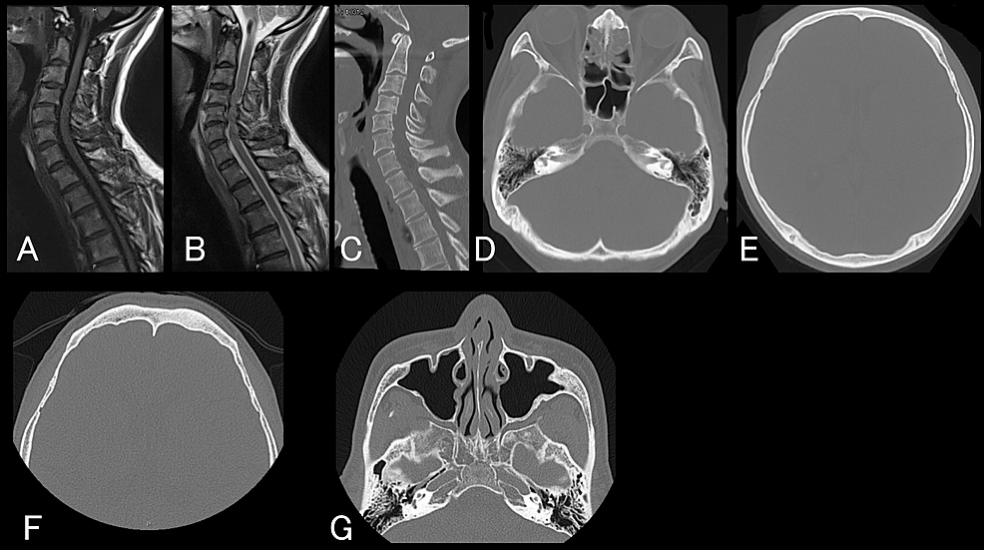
Images at the time of trauma and one year from the trauma Upper row (images at the time of trauma): T1-weighted (A) and T2-weighted (B) magnetic resonance imaging. They revealed cervical spinal cord injury without cervical vertebral fracture (C). Head computed tomography (CT) images did not suggest cranial fracture nor pneumocephalus (D, E). Lower row: CT images still did not reveal a fracture, fistula, pneumocephalus, nor injury of the dura (F, G).
